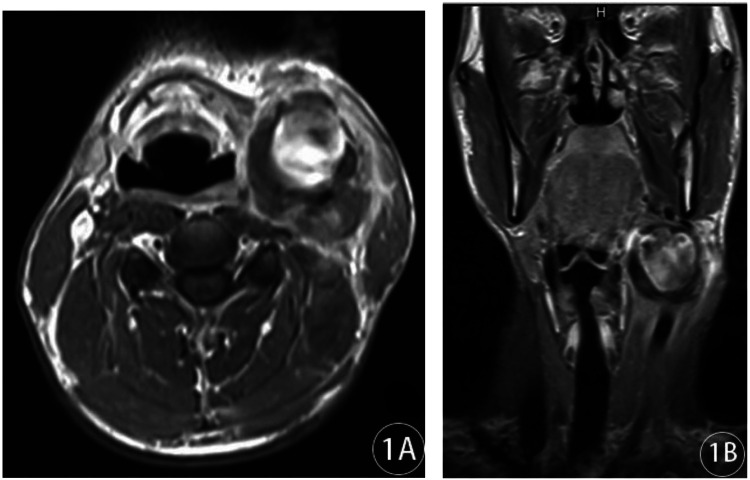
Plain MRI + enhanced MRI of the neck: (A) axial and (B) coronal.
